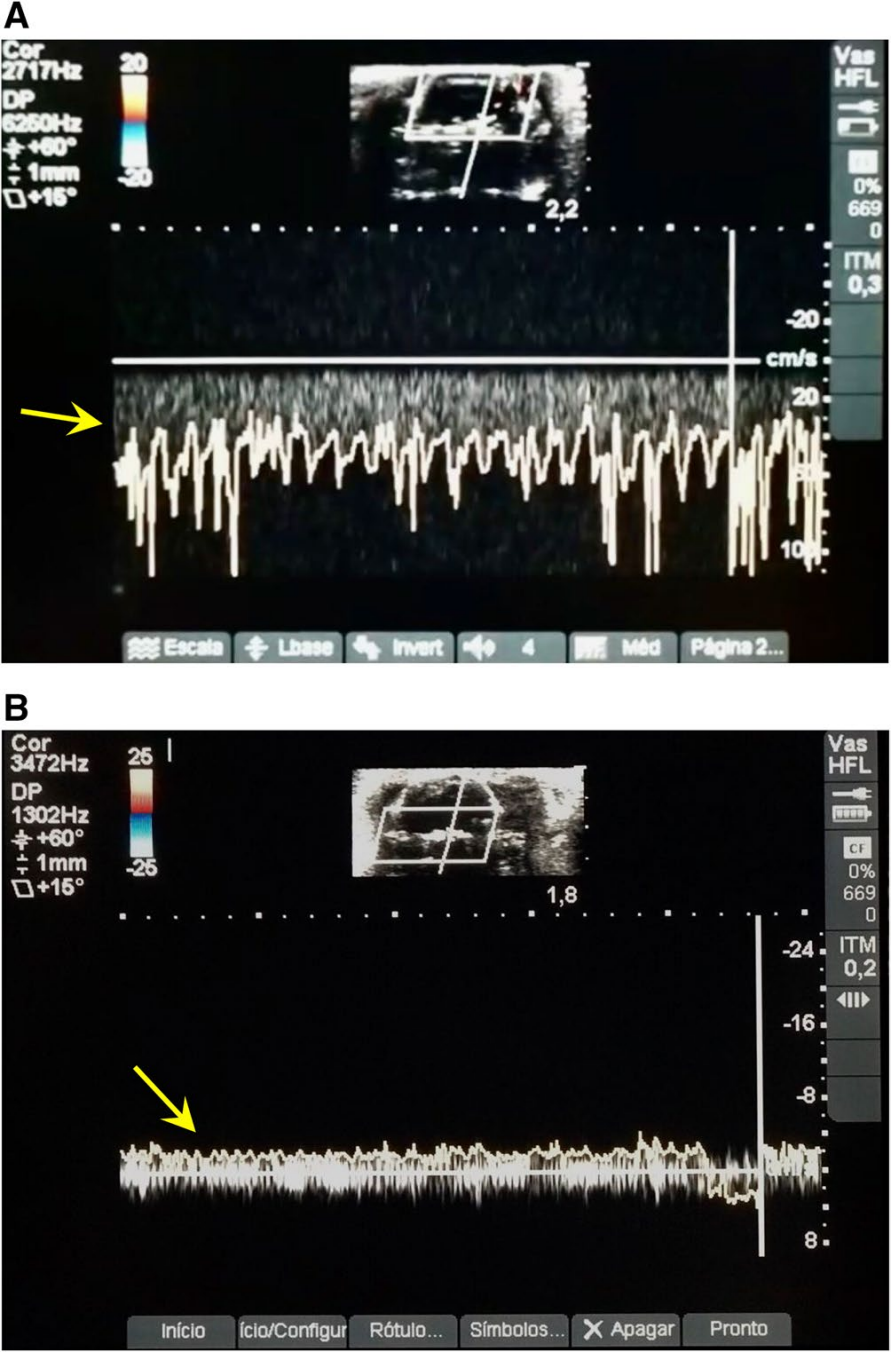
Ultrasound imaging and flow changes of the cerebral arteries measured in a cross-sectional direction. (A) Ultrasound imaging assessments before brain death induction. Note the presence of blood flow in the reverse direction (arrow). (B) Ultrasound imaging assessments 6 h after brain death induction. Note the blood flow compatible with brain blood circulatory collapse (arrow).
